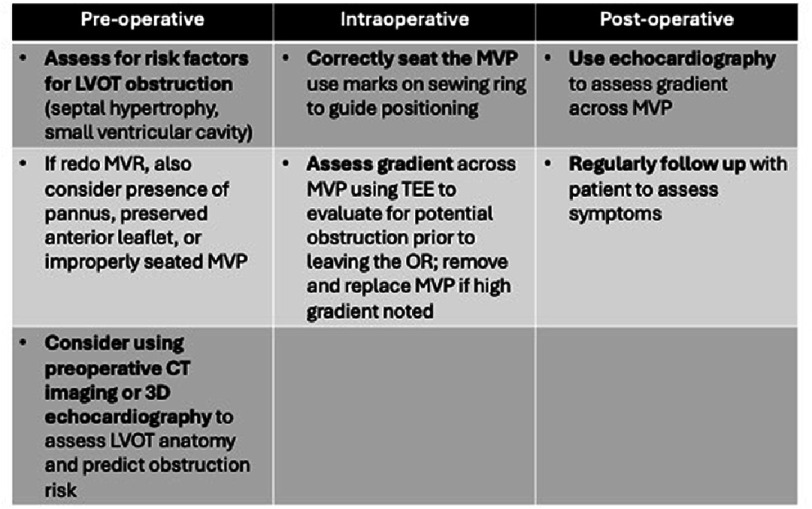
Decision making framework and important considerations for trainees to prevent LVOT obstruction in MVR. The framework has separated into pre-operative, intraoperative, and post-operative stages to help organize important considerations. CT, computed tomography; LVOT, left ventricular outflow tract; MVP, mitral valve prosthesis; MVR, mitral valve replacement; OR, operating room; TEE, transesophageal echocardiography.
